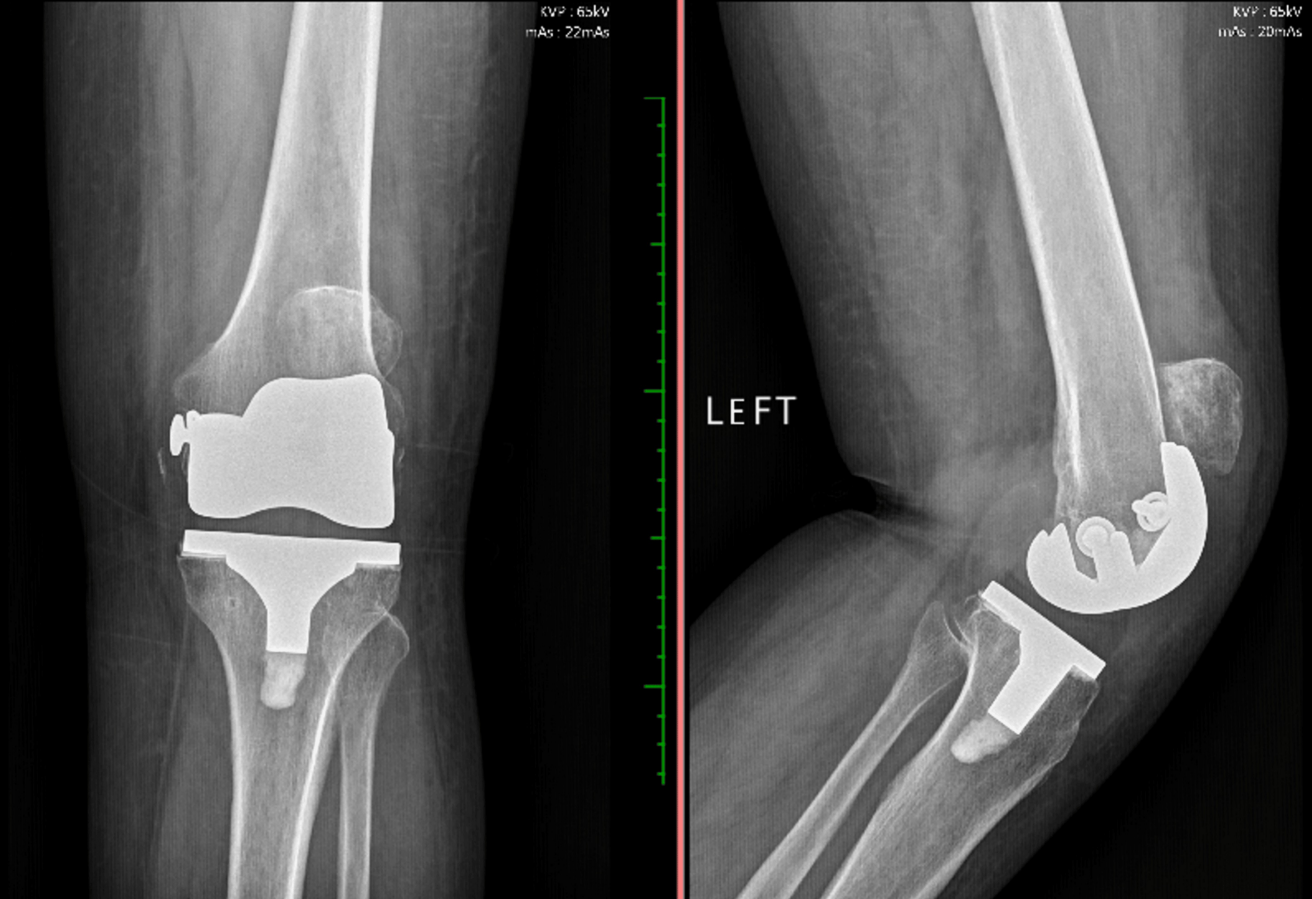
Knee radiograph in AP and lateral views, showing patellar elevation. AP: anteroposterior
